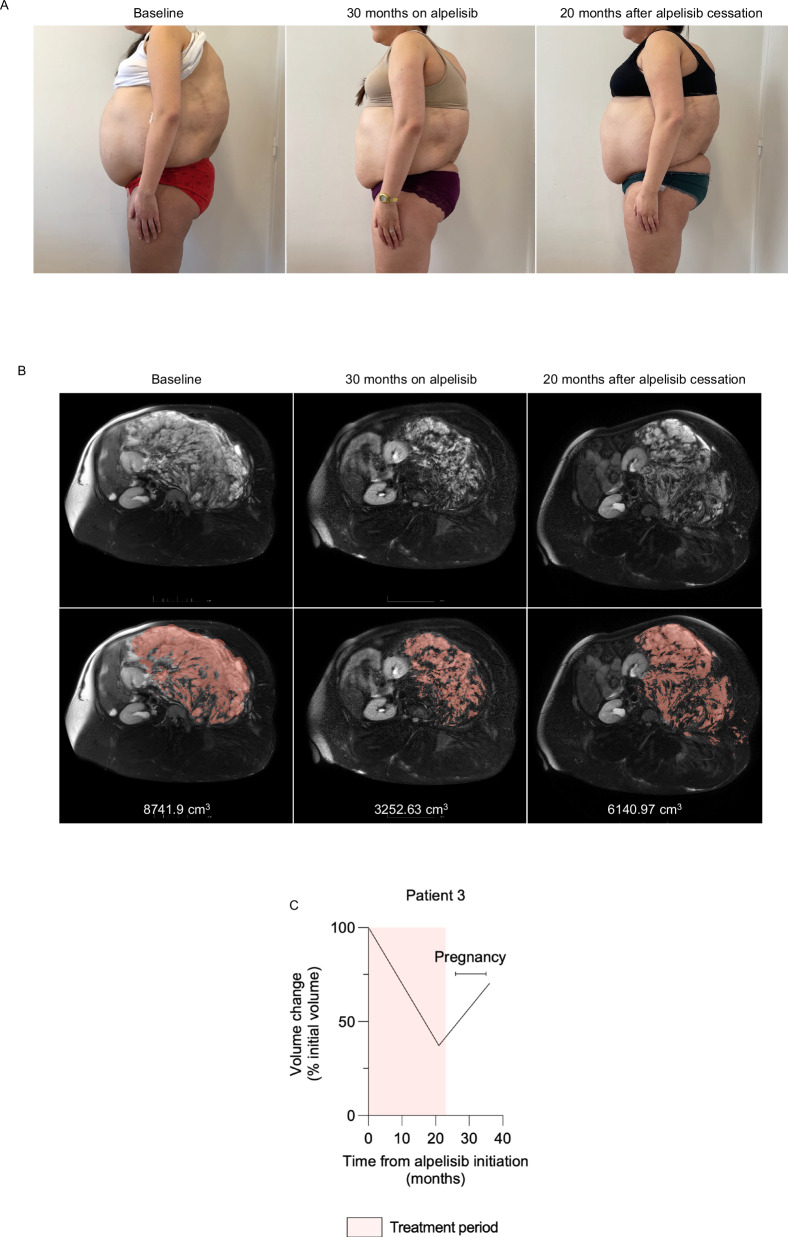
Patient 3 following alpelisib withdrawal and reintroduction. A Representative pictures of patient 3 over time. B Representative transversal MRI T2 fat sat sequences of patient 3 over time. C Magnetic resonance volumetric of the malformation over time.
